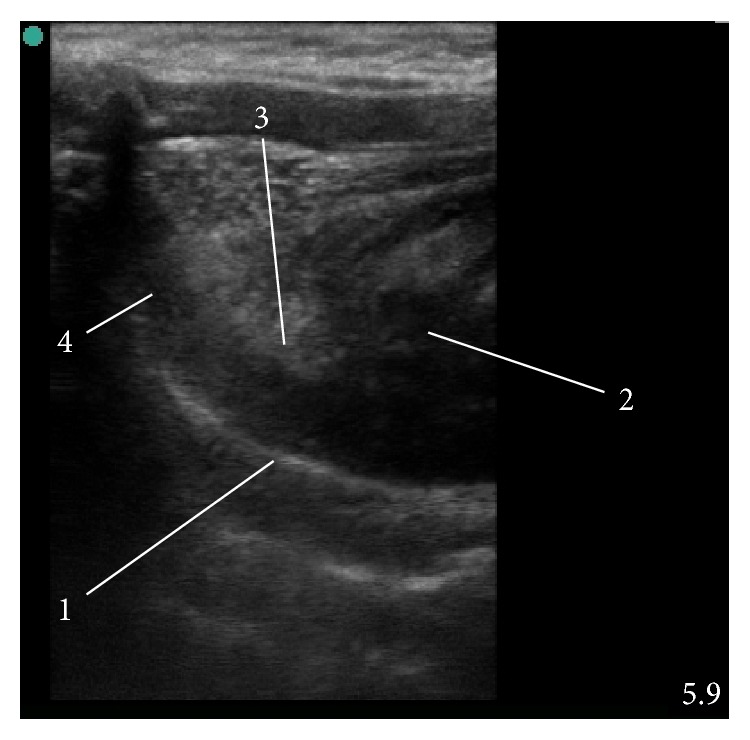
Ultrasound visualization of the tongue and floor of the mouth. (1) Hard palate, (2) tongue, (3) epiglottis, and (4) laryngeal inlet. The picture is “upside down” because the examination is performed via the sublingual window and therefore the hard palate is the deepest structure on the image.
